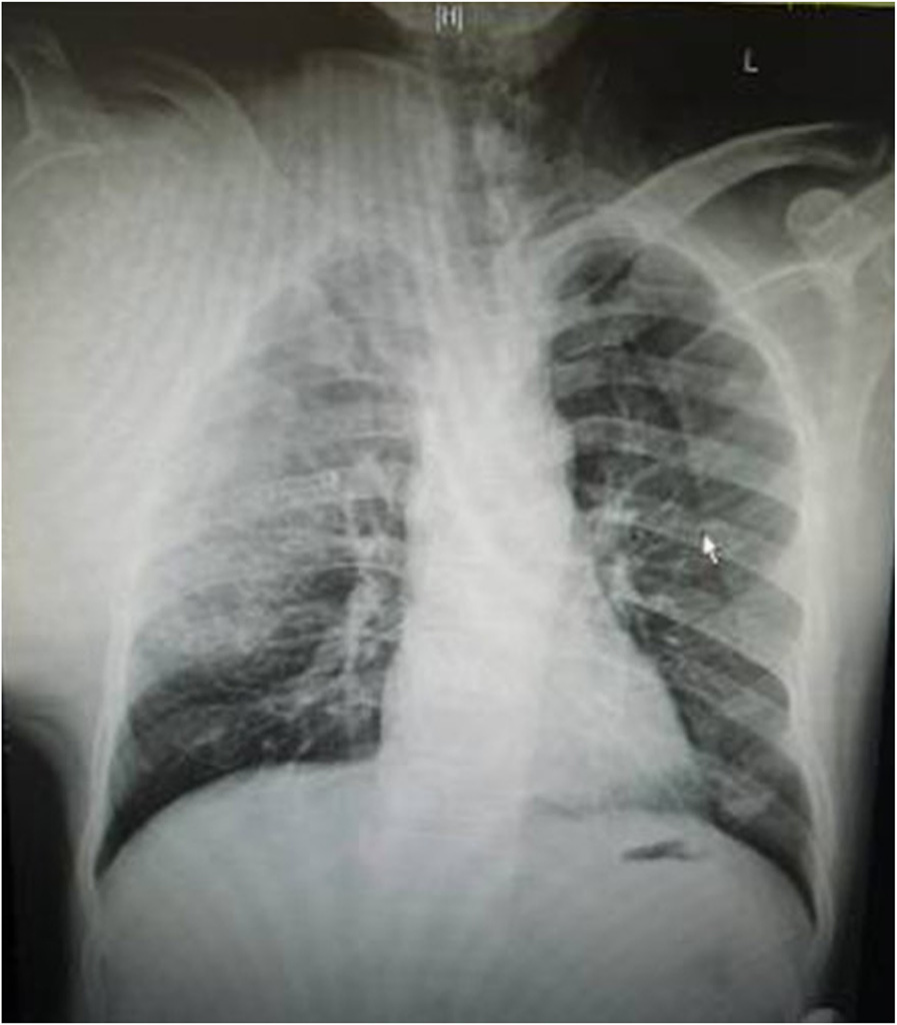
The radiograph of the right shoulder showing the tumor in the right scapula before surgery.
